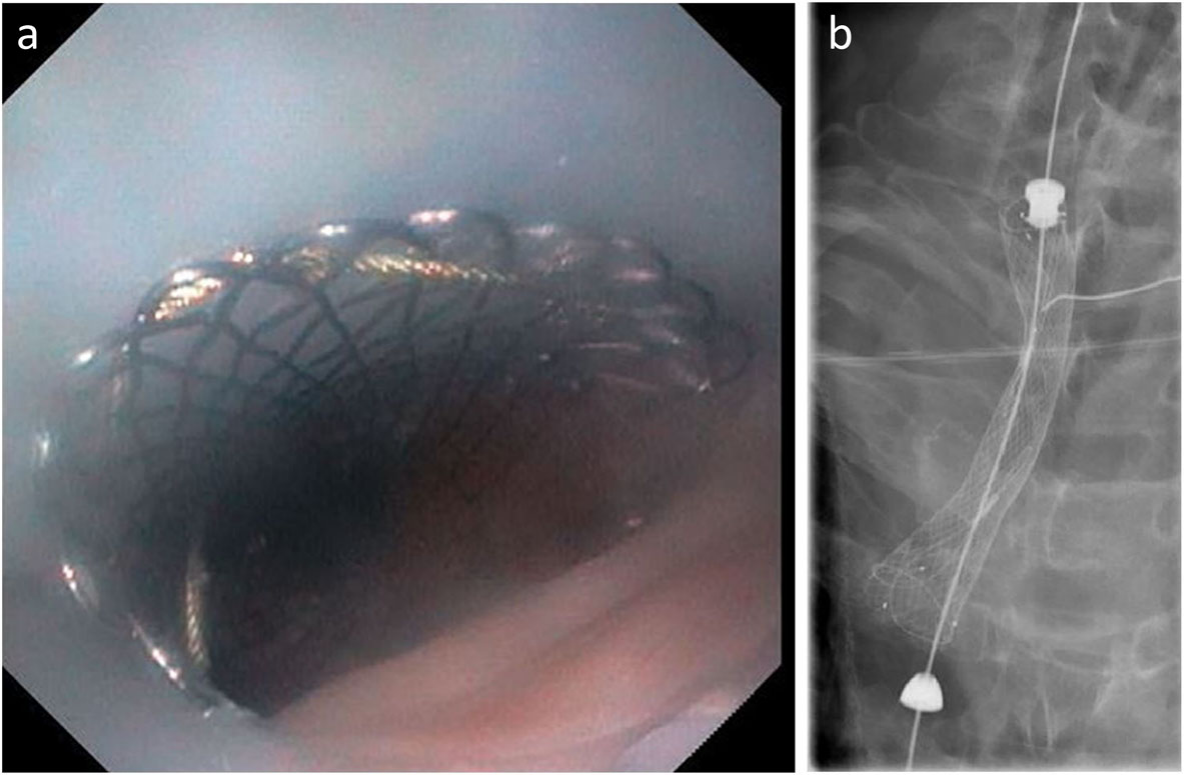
Esophageal stent placement. a Endoscopic view through the gastric channel of a LMA Gastro™. b Chest X-ray: Esophageal stent placement, LMA Gastro™ in situ
